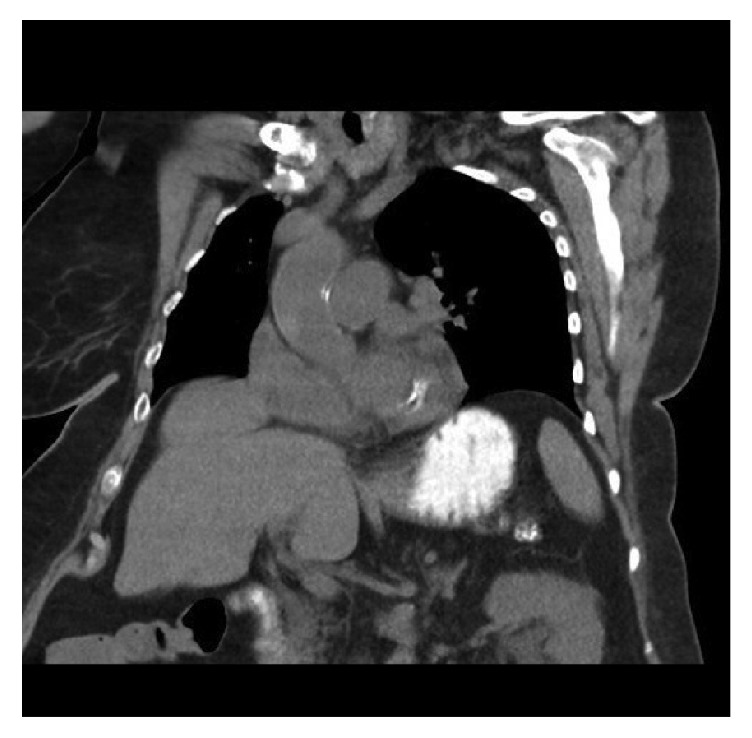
Sagittal CT scan demonstrating no free fluid in the abdomen.
